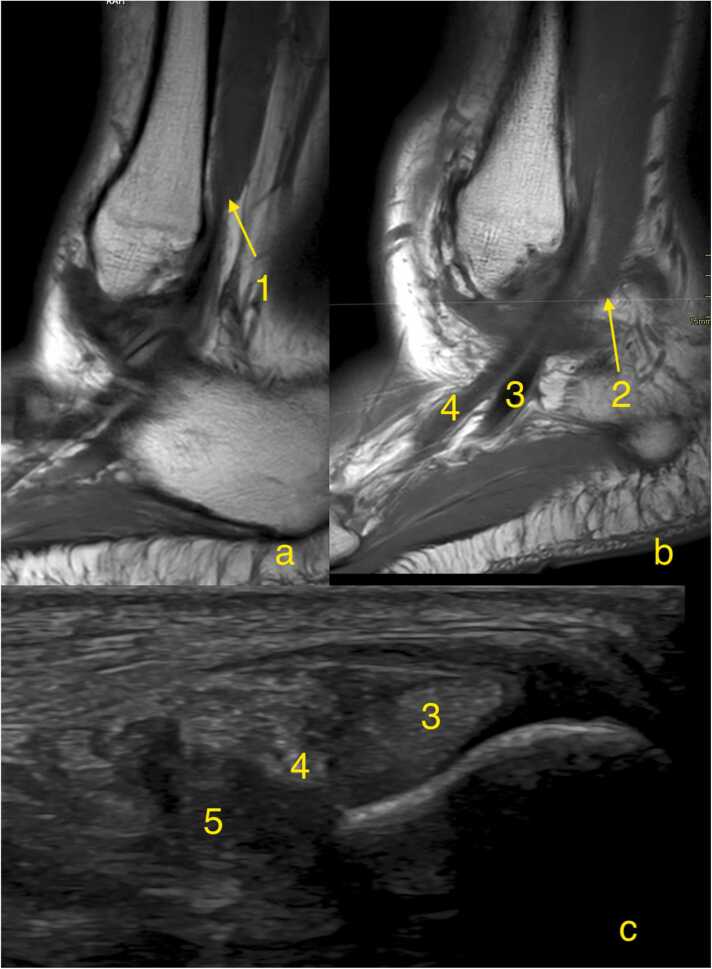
Anatomical variations of the peroneus brevis muscle in three different patients. (a, b) Sagittal cross section, T1-weighted images, (c) Transverse cross section ultrasound. 1- Muscle belly ending superior to the lateral malleolus, 2- Muscle belly ending at the tip of the lateral malleolus, considered a low-lying muscle belly, 3- Peroneus longus, 4- Peroneus brevis, 5- Muscle belly found in the superior peroneal tunnel, considered a low-lying muscle belly.
